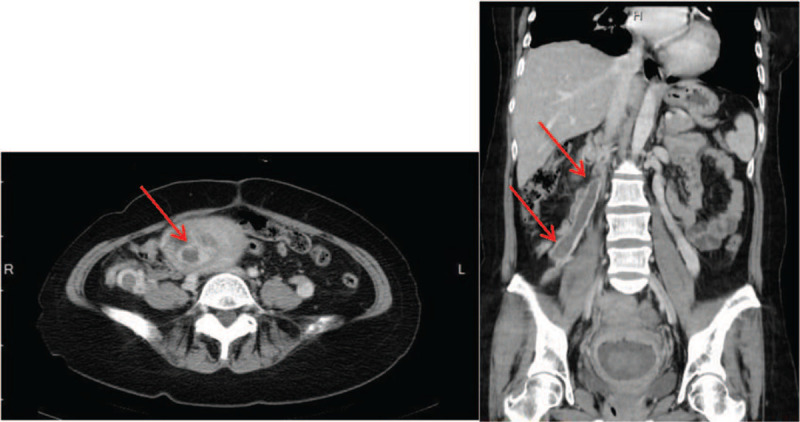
Contrast-enhanced computed tomography scan of the patient.
